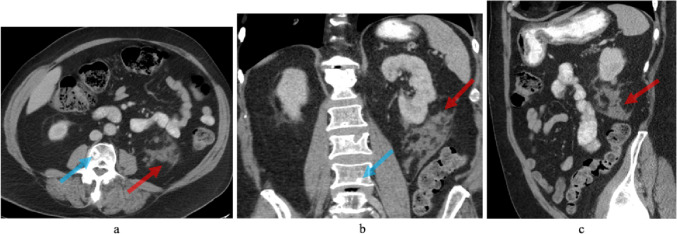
Retroperitoneal Angiosarcoma. Axial (a), coronal (b) and sagittal (c) contrast-enhanced CT images demonstrate multiple ill-defined enhancing soft tissue nodules in the left inferior perinephric space (red arrows) due to biopsy-proven angiosarcoma. The patient also had a history of prostate cancer with bony metastatic disease (blue arrow)
